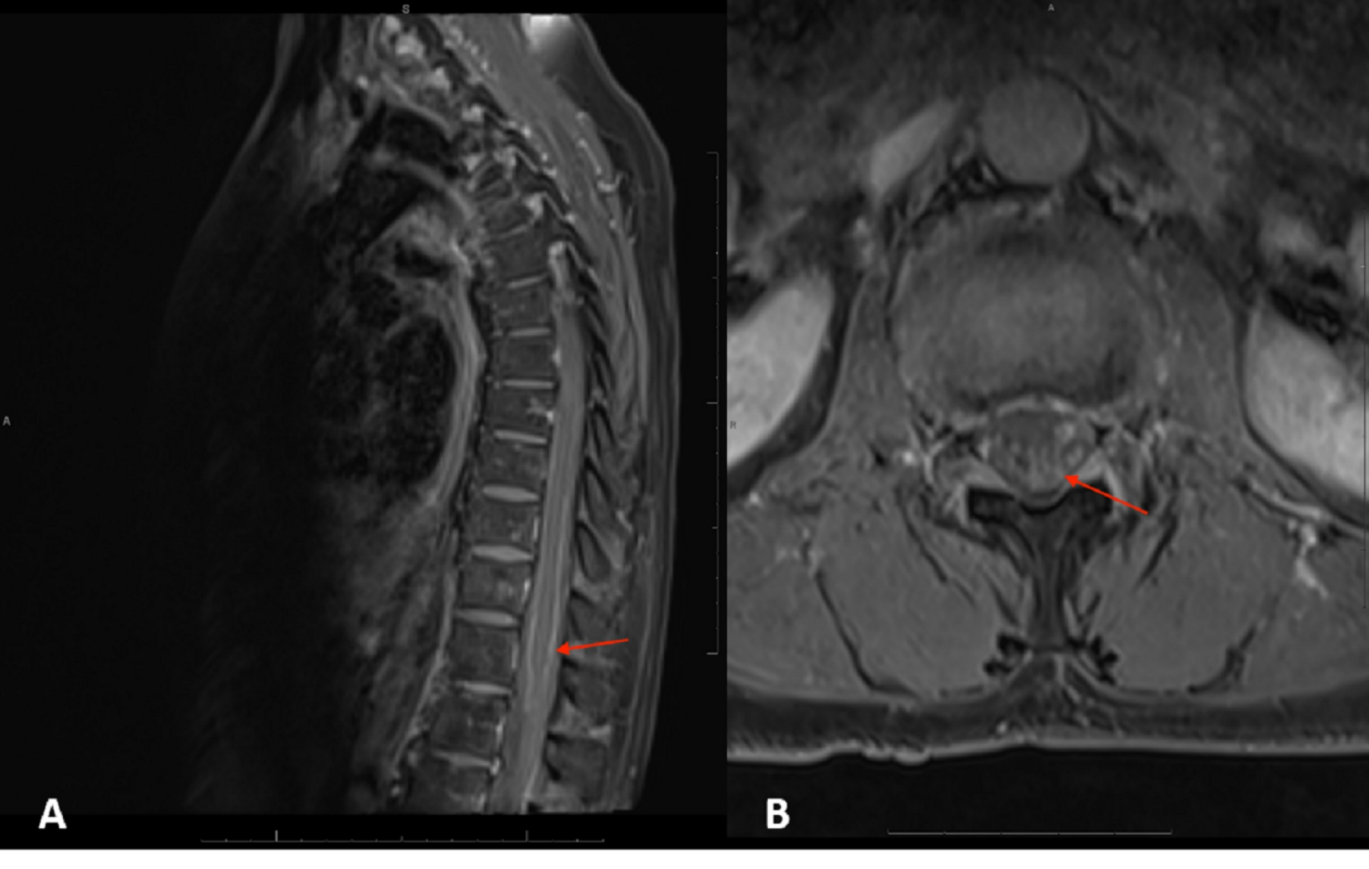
T1-weighted magnetic resonance imaging (MRI) with contrast and fat suppression of the spine that was interpreted as arachnoiditis (A) Sagittal view of lumbar spine; (B) L4-L5 nerve root clumping was the only suspicious sign for leptomeningeal carcinomatosis (LMC) on MRI
